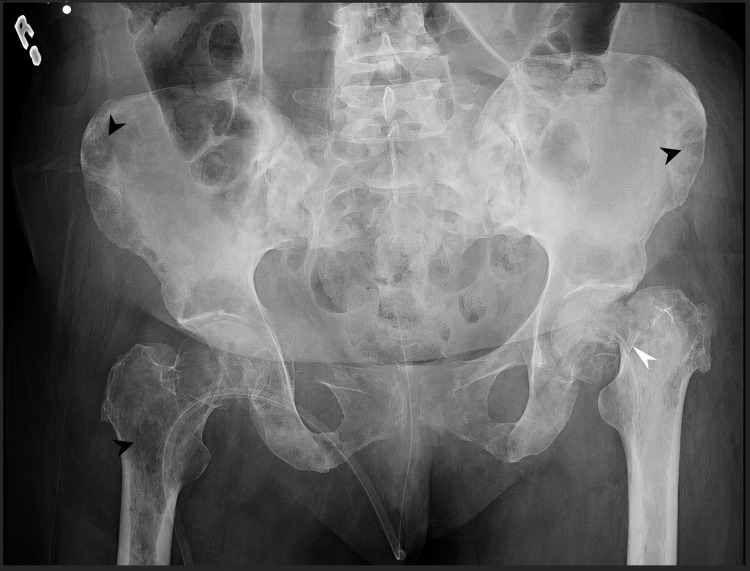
Plain X-ray of the pelvis showing multiple innumerable lytic lesions involving the whole pelvic bones (black arrowhead). A pathological fracture of the left femur neck is noted (white arrowhead).
